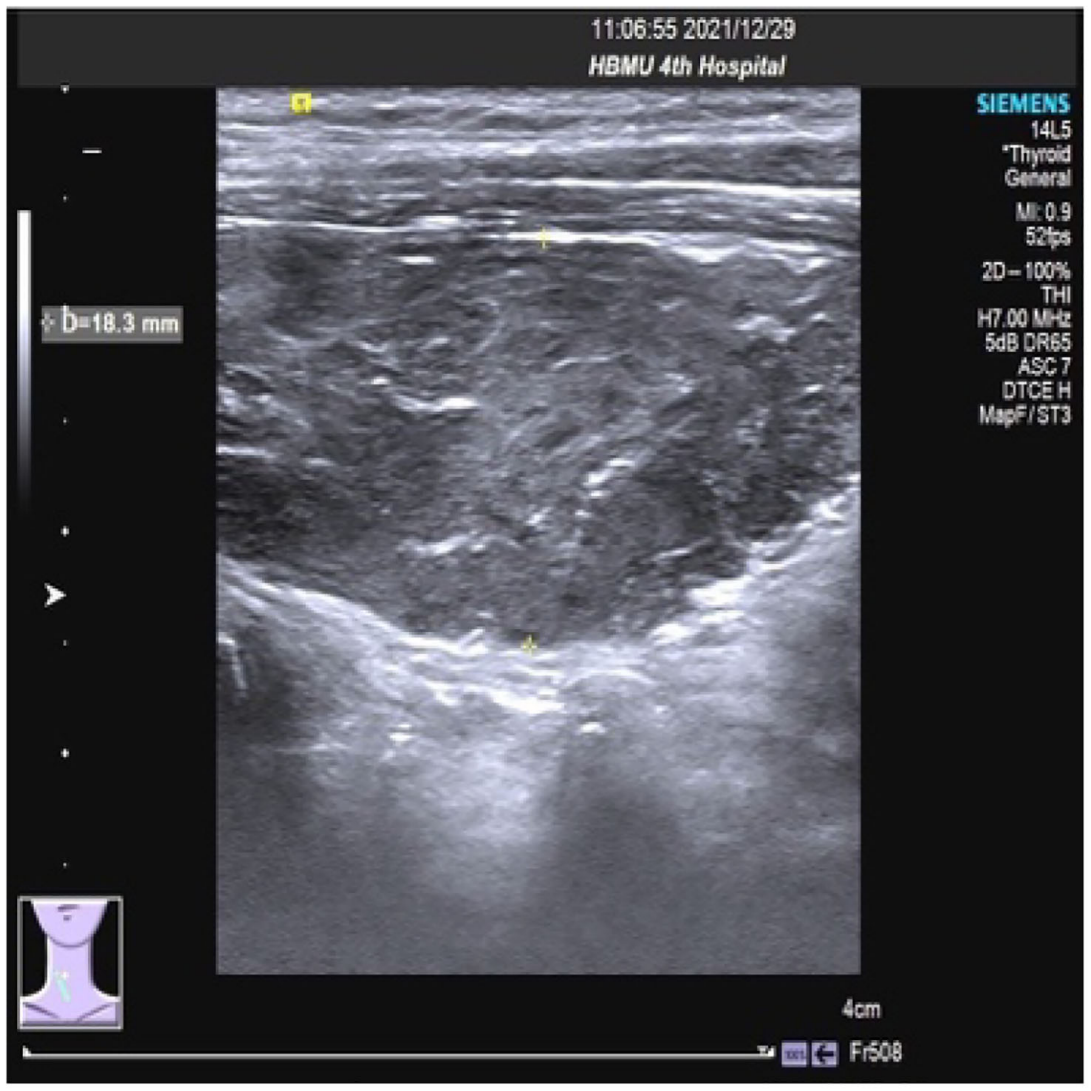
Thyroid ultrasound results showing diffuse thyroid lesions.
